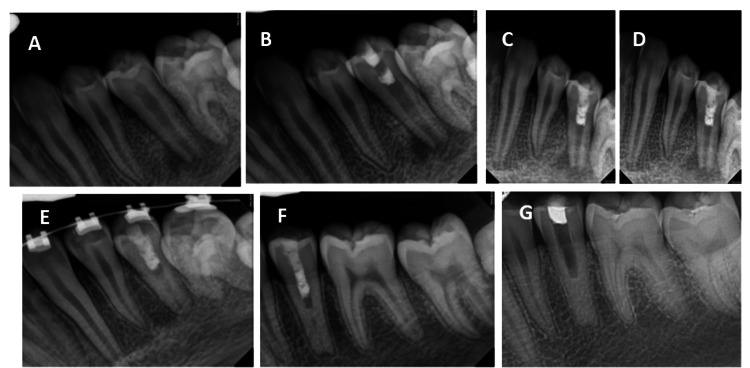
Case 2 - radiographic images (A) Preoperative radiograph (October 2012) showing tooth #20 with an open apex and periapical radiolucency. (B) Radiograph after the initial regenerative endodontic procedure showing placement of mineral trioxide aggregate and CollaCote barrier (OsseoDent, Miami, USA). (C) Follow-up radiograph at 4 months (February 2013) showing persistent periapical pathology prompting retreatment. (D) Radiograph obtained on the same day showing completed repeat regenerative endodontic procedure using mineral trioxide aggregate and CollaCote barrier. (E) Recall visit radiograph at 10 months showing thickening of dentinal walls and an increase in root length. (F) Recall visit radiograph at 14 months showing increased calcification of the apical half of the canal space and normal root length. (G) Radiograph showing an empty canal space with temporary restoration.
